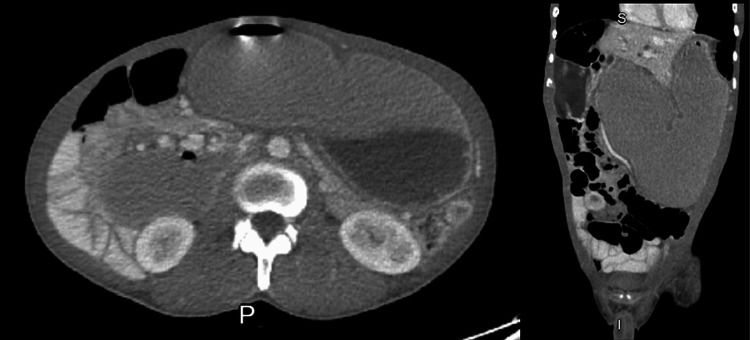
CT abdomen showing extensive abdominal dilatation.
